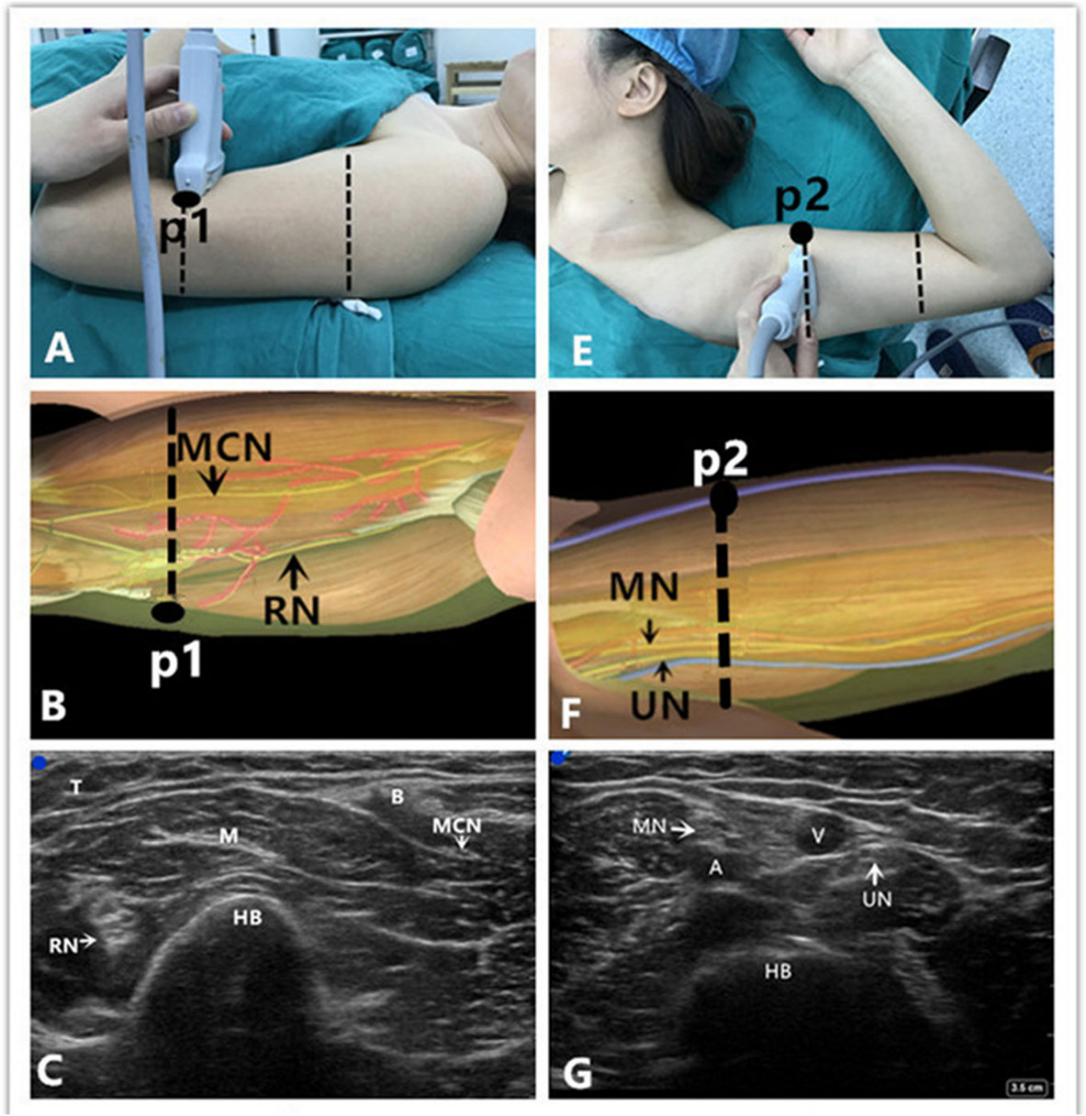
Position of patient, neuroanatomy and ultrasound imaging at the level of upper-median 1/3 and low-median 1/3 of the arm in the operating room. a Position of the patient, the probe, and the needle (“p1”) during block of the radial and musculocutaneous nerve. b Topography of the radial nerve (RN) and the musculocutaneous nerve (MCN); red = muscular branches; and the black point “p1”denotes the puncture site. The dotted line denotes the probe position. c Ultrasound depiction of the radial nerve (RN) and the musculocutaneous nerve (MCN). The blue point is situated at the lateral side of the probe. The RN is round with a hyperechoic structure and is located between brachialis and humerus laterally. The MCN is fusiform with a hyperechoic structure, and is located between biceps and brachialis close medially. Also note the humerus (HB), and the triceps (T), biceps (B), and brachialis (M) muscles. e Position of the patient, the probe, and the needle (“p2”) during block of the median, ulnar, and medial antebrachial cutaneous nerves. f Topography of the median nerve (MN), the ulnar nerve (UN). The black point “p2”denotes the puncture site. The dotted line denotes the probe position. g Ultrasound description of the median nerve (MN) and the ulnar nerve (UN). The blue point is situated at the cephalic side of the probe. The MN is between 12 and 1 o’clock in relation to the humeral artery. The UN is situated at the 3 o’clock position of the basilic vein, brachial artery (A), basilic vein (V), and humerus (HB)
